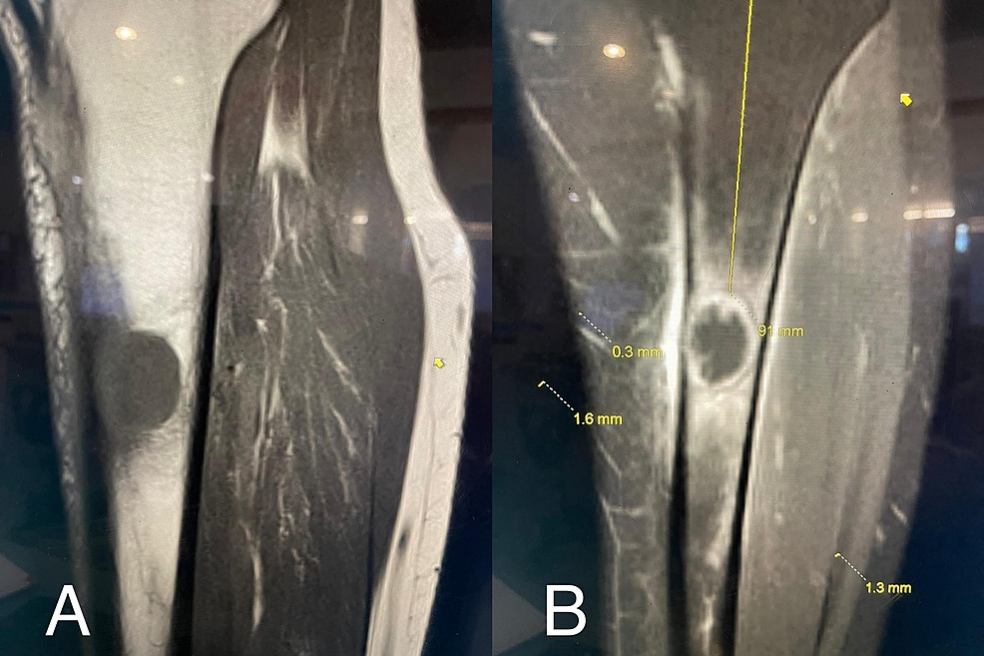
Magnetic resonance imaging (MRI) of the left tibia showing sagittal and coronal views (A) Sagittal view and (B) coronal view of the left tibia, confirming an osteolytic bony lesion noted in the upper shaft of the left tibia and with its superior aspect. Appearances are suggestive of an aggressive bony lesion.
